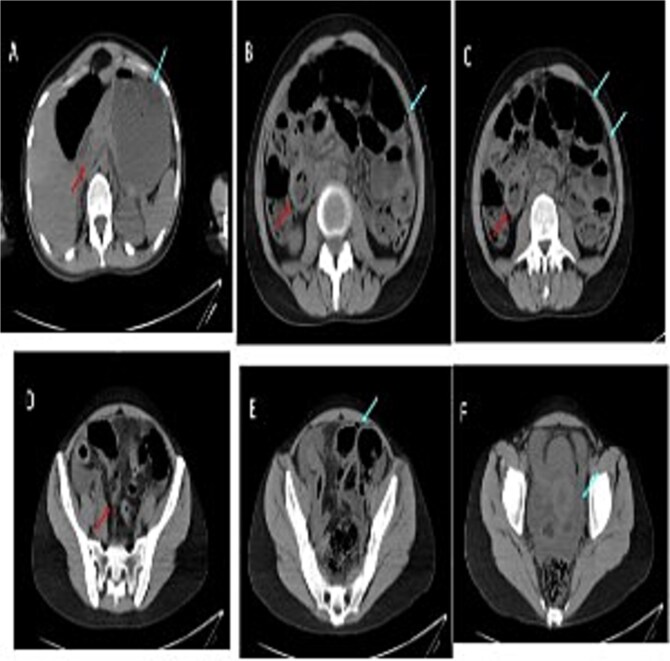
Serial images from the patient computed tomography abdominal scan that showed terminal ileum wall thickening contributing to luminal narrowing, along with surrounding fat stranding and mesenteric congestion which is associated with some adjacent prominent lymph nodes. There is few foci of extraluminal free gas reflecting bowel perforation, in addition to free fluid in the pelvis and paracolic gutters. Despite the presence of some bowel dilatation no complete bowel obstruction and the rectum lumen is preserved.
